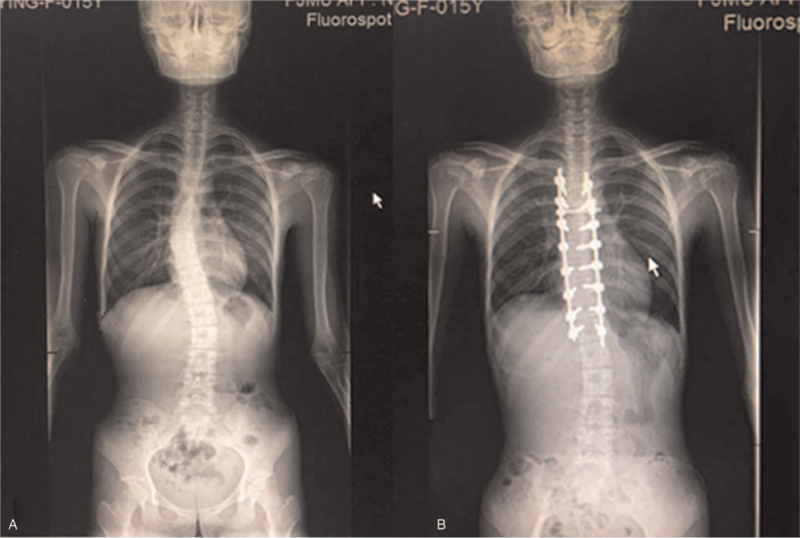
A, Preoperative spinal X-ray film. B, Postoperative spinal X-ray film.
